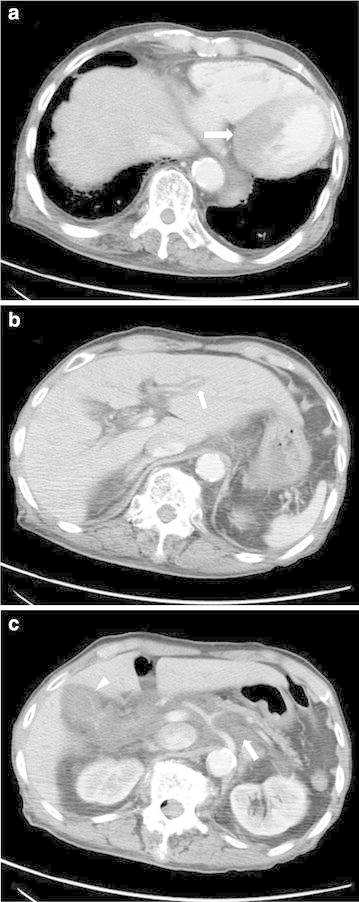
Axial contrast-enhanced computed tomography at the level of the heart (a), left portal vein of the liver (b), and pancreatic body (c). Part of the left ventricular wall (*arrow*) is not enhanced in (a). The periportal collar sign (*arrow*) is depicted in (b). Lymphedema in the anterior pararenal space (*arrow*) and subserosal edema of the gallbladder wall (*arrowhead*) are depicted in (c).
